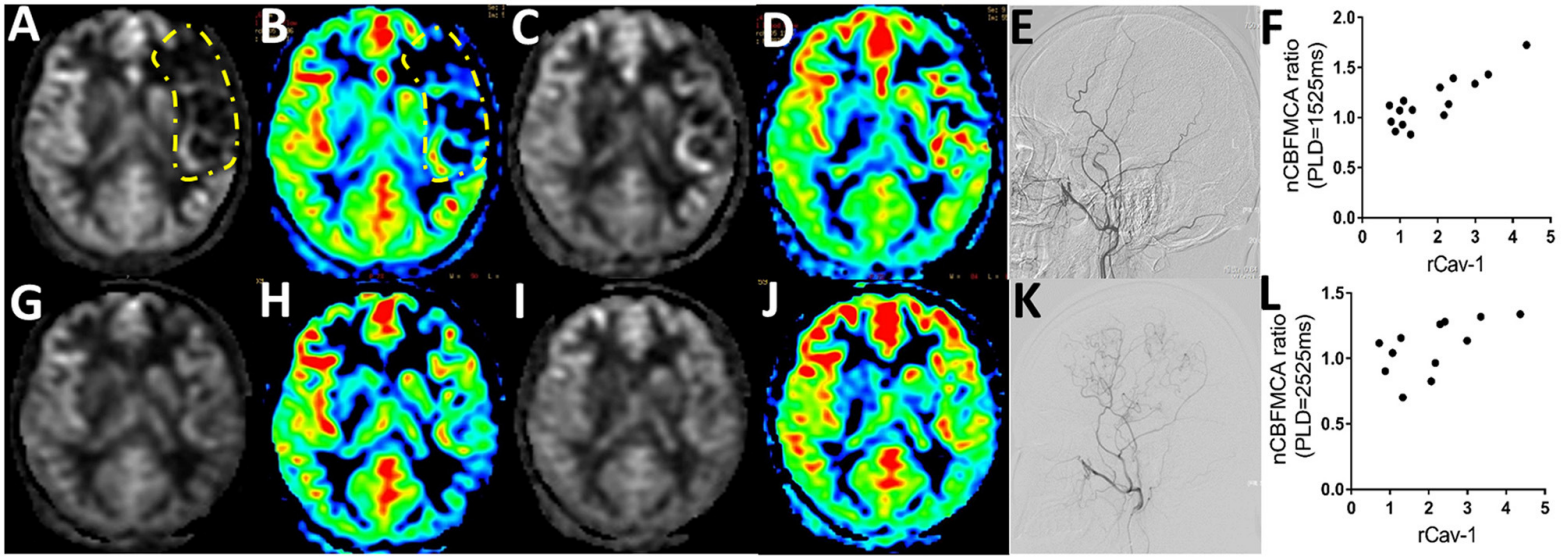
Serum Cav-1 was associated with cerebral blood flow (CBF) determined by arterial spin labeling MRI (ASL-MRI). (A) 24-year-old female patient was hospitalized for right hand numbness and diagnosed as MMD. The raw and color preoperative CBF maps by regular postlabeling delay (PLD) (A,B) and long PLD pseudocontinuous ASL (pCASL) imaging (C,D) demonstrated the hypoperfusion of middle cerebral artery (MCA) territory (circled by yellow lines). The CBF improved obviously in 6 months postoperative pCASL scanning with regular PLD (G,H) and long PLD (I,J). The collaterals from the left external carotid artery before and after bypass surgery were shown in (E,K) by DSA. The rCav-1 had a strong positive relationship with the nCBF_MCA_ ratio in both the regular (*r* = 0.8615, *p* < 0.0001, (F)) and long PLD (*r* = 0.5915, *p* = 0.0428, (L)) by Spearman analysis. nCBF_MCA_ ratio, postoperative/preoperative nCBF_MCA_; nCBF_MCA_, CBF of MCA territory/CBF of ipsilateral cerebellum; rCav-1, postoperative/preoperative Cav-1.
